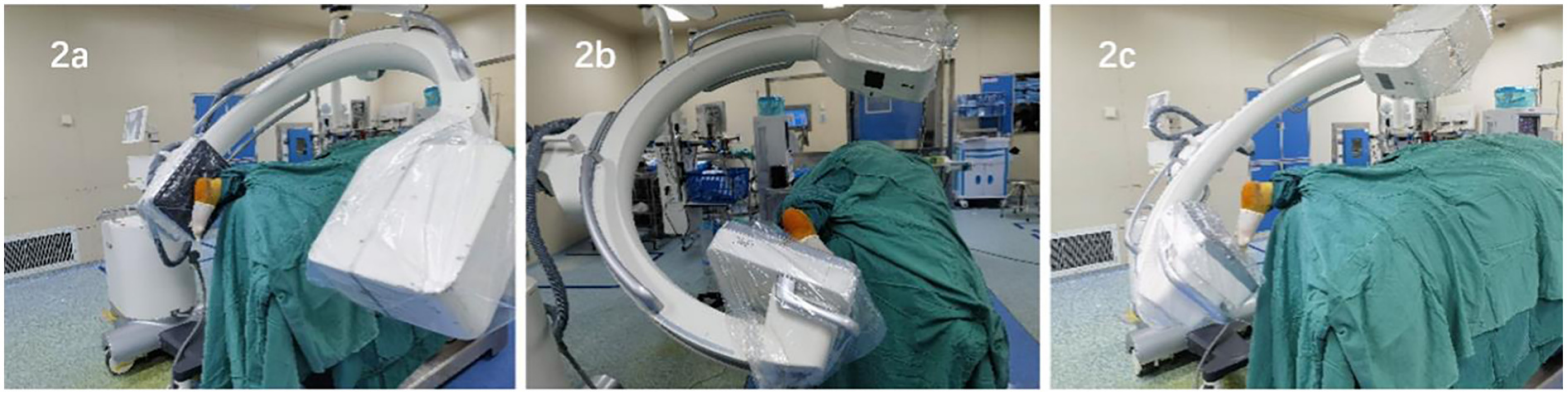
Photos pf patient positioning and placement of the C-arm x-ray machine (a) lateral view of the calcaneus under fluoroscopy, (b) broden view of the calcaneus under fluoroscopy, (c) axial view of the calcaneus under fluoroscopy.
